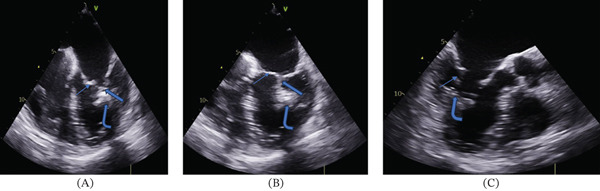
Transesophageal echocardiography apical four chamber (A, B) and three chamber (C) views revealed a calcified anterolateral papillary muscle (curved arrow). The enlarged and extended chordae merged into a single papillary muscle (arrow). Additionally, dysplastic chordae tendineae and thickened mitral valve leaflets (narrow arrow) were observed in the four‐chamber view.
